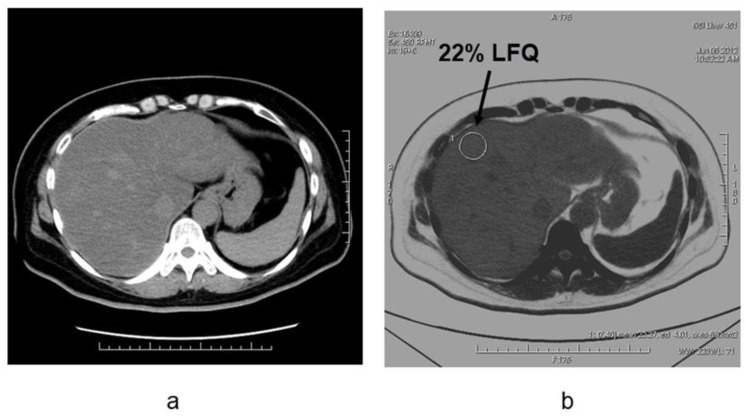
Liver fat measurements by using dual-energy CT in the patient with NAFLD (non-alcoholic fatty liver disease)(a) Noncontrast CT image and (b) fat density image in patients with nonalcoholic fatty liver disease. The degree of fat deposition was quantifiable on the fat density images, and the quantitative assessment of liver tissue and fat in this case was 22% liver-fat quantification.
